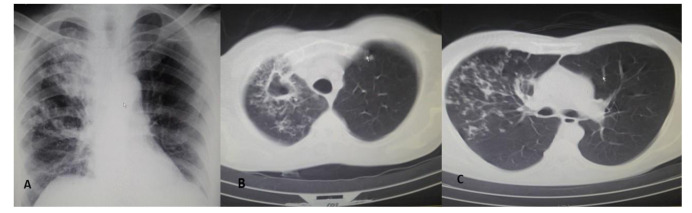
Chest radiography of posterior-anterior projection (view) and thorax computed tomography A. Chest X-ray, posterior-anterior projection, right upper lung infiltration upon admission B. Computed tomography of the right lung cavitary lesions in the right lung C. Computed tomography of the right lower lung showing tree-in-bud infiltration infiltrations
